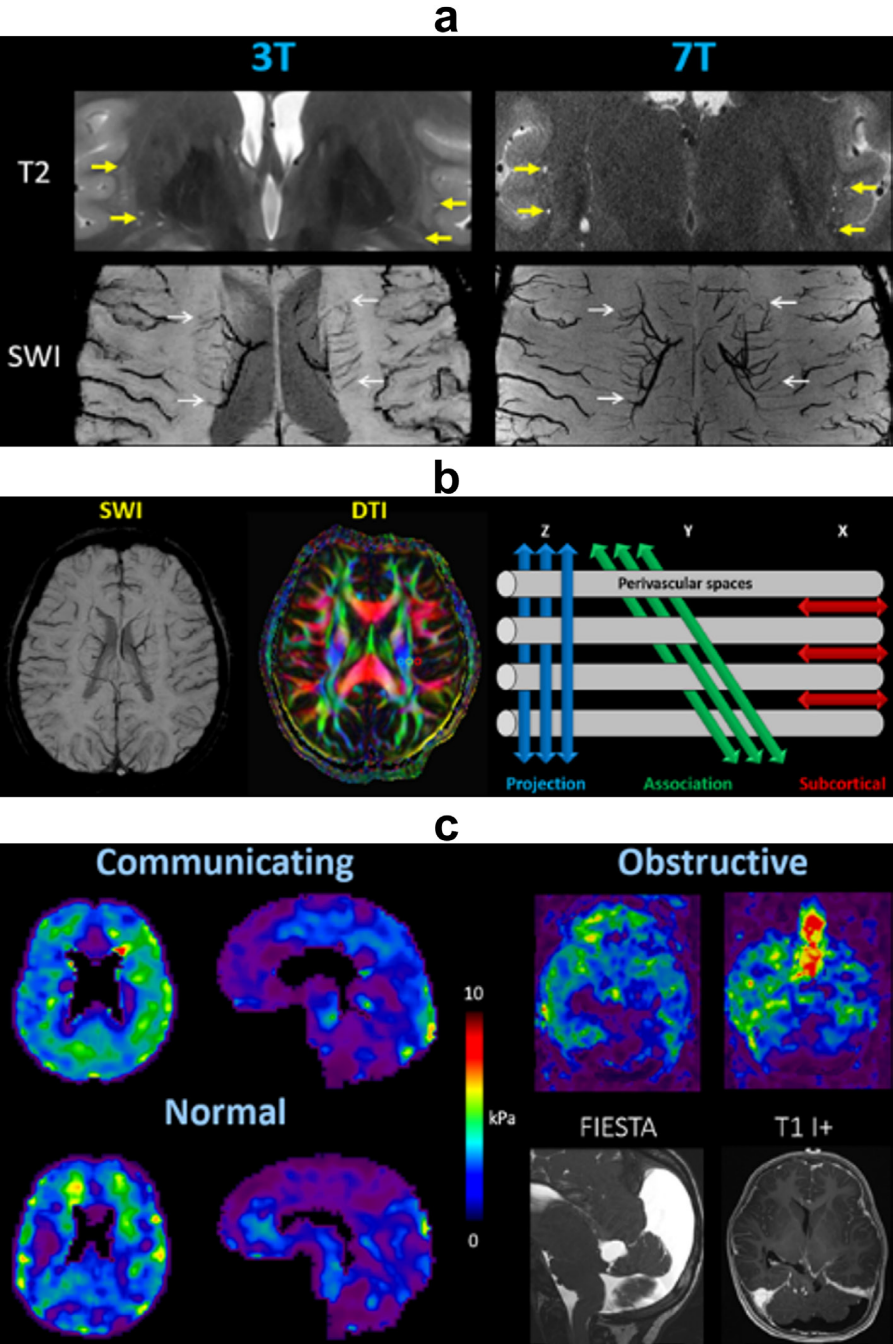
Structural imaging. (a) PVS are better delineated on ultra-high-field (7 Tesla) MRI compared to 3 Tesla, due to the higher SNR and spatial resolution. PVS appear as CSF-filled spaces (thick yellow arrows) with hyperintense signal on T2-weighted imaging, and surround penetrating small vessels (thin white arrows) with hypointense signal on SWI. (b) DTI-ALPS index. Blue: projection fibers of corticospinal tract in Z-axis (superior–inferior), green: association fibers of superior longitudinal fasciculus in Y-axis (anterior–posterior), red: subcortical fibers of subcortical U tracts in X-axis (left–right). The ALPS index is computed as the average diffusivity along the PVS (X-direction) divided by average diffusivity in perpendicular white matter tracts (Y- and Z-directions). (c) Magnetic resonance elastography shows stiffer brain parenchyma in communicating hydrocephalus and obstructive hydrocephalus compared to normal controls. ALPS, ALong the Perivascular Spaces; CSF, cerebrospinal fluid; DTI, diffusion tensor imaging; FIESTA, fast imaging employing steady-state acquisition; kPa, kilopascals; PVS, perivascular spaces; SWI, susceptibility-weighted imaging.
